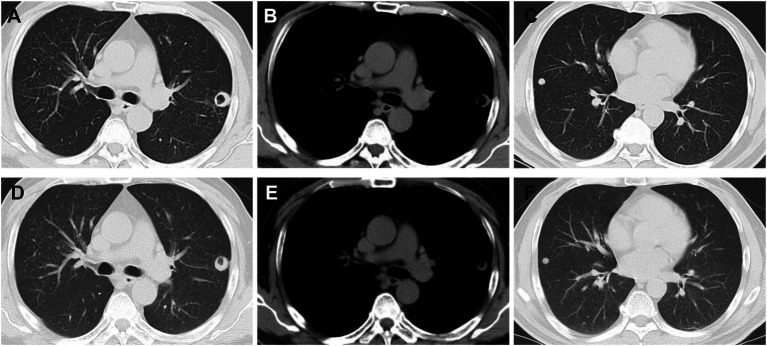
(A–C) CT shows miliary nodular opacities with cavitary evolution in one lesion (Series 301/Image 26), approximately 16 × 15 mm. (D–F) CT shows miliary nodular opacities with cavitary evolution in one lesion (Series 201/Image 25), approximately 16 × 14 mm.
